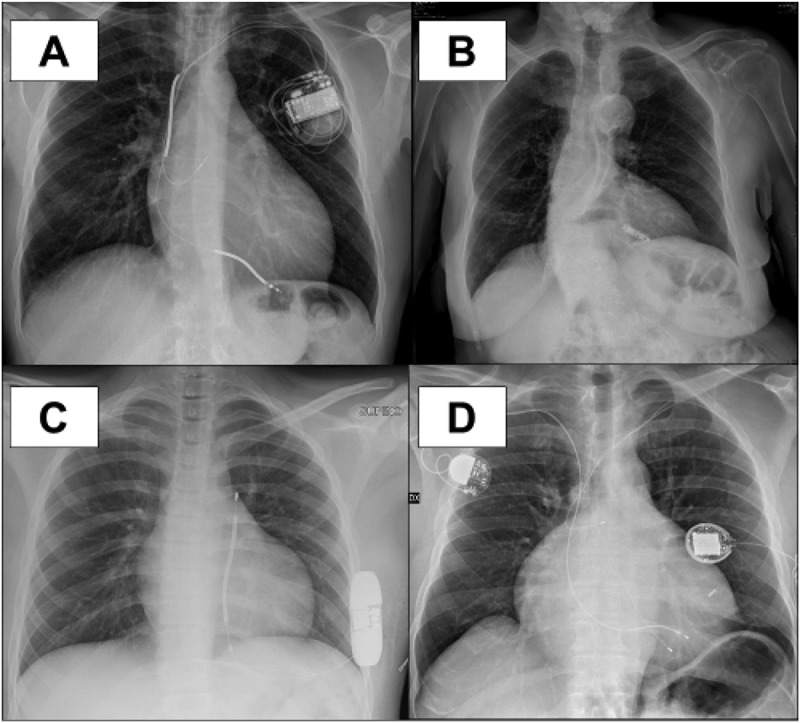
Chest X rays showing different types of implanted electrical devices that can be considered as current therapeutic options, according to the indications of consensus guidelines. A: transvenous cardioverter-defibrillator for prevention of sudden arrhythmic death; B: leadless VVI pacemaker; C: subcutaneous implantable cardioverter defibrillator; D: leadless ultrasound-based left ventricular pacing for cardiac resynchronization therapy in combination with a conventional pacemaker positioned in the right infraclavicular region.
